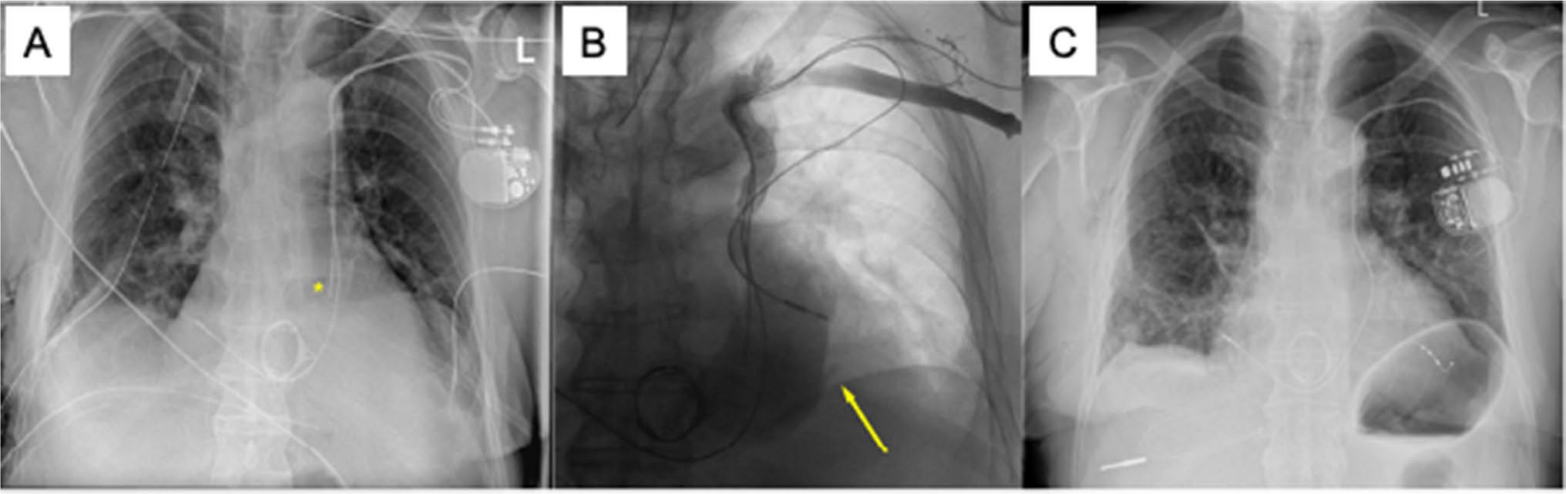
Case # 5: chest X-ray after tricuspid valve replacement with the asterisk at the end of the stripped-down right ventricular lead (A). Initial angiography showing PLSVC and the ostium of a posterolateral CS branch (yellow arrow) (B). Final chest X-ray on first postoperative day (C)
